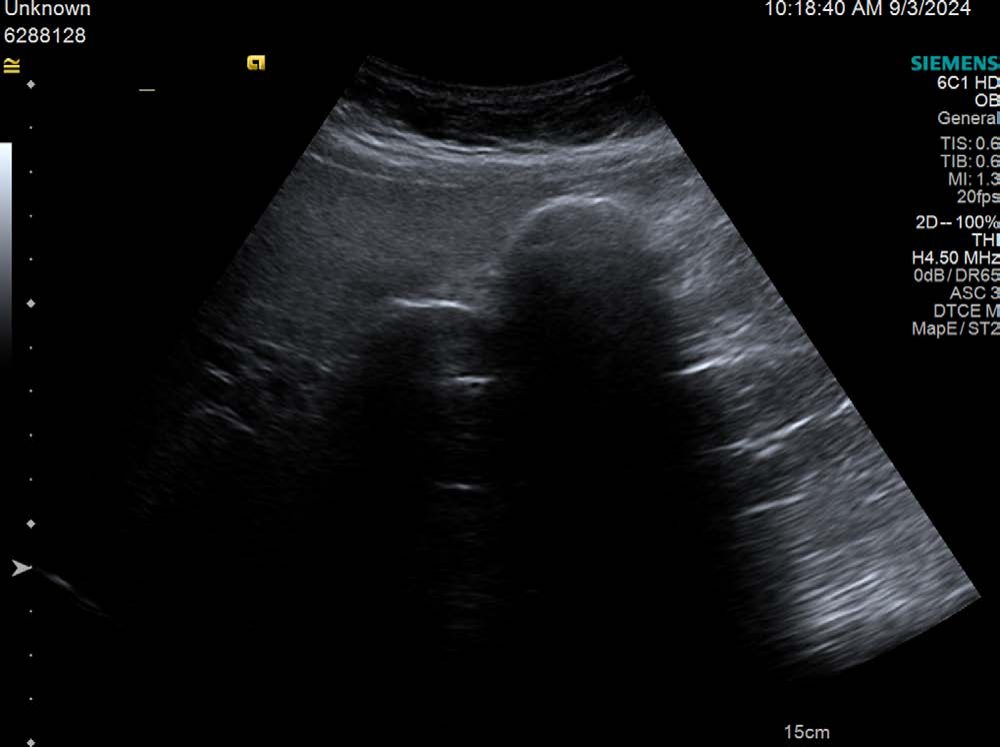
Apart from full-filled gallstones, abdominal ultrasonography also showed an obscure gallbladder wall with meniscus-shaped strong echo.
